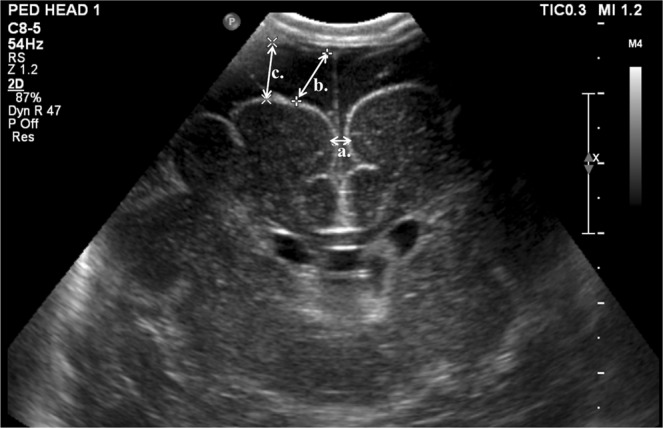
An example of cranial ultrasound image of the subarachnoid space captured via the coronal view ((a) IHW, interhemispheric width; (b) SCW, sinocortical width; (c) CCW, craniocortical width).
